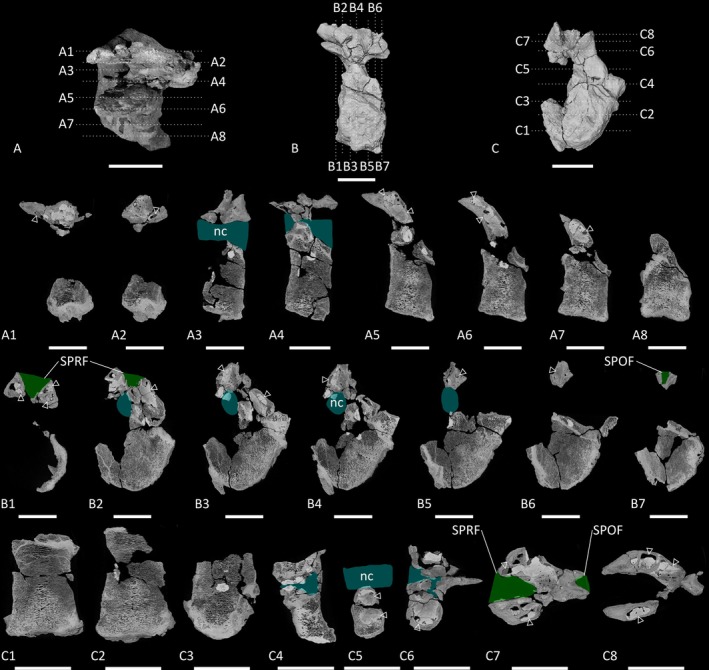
CT scan of *Wintonotitan wattsi* anterior caudal vertebra ‘D’ QMF7292. (A) photograph dorsal view, (B) 3D rendering left lateral view, (C) 3D rendering anterior view; (A1–A8) CT scan slices in parasagittal cross‐section, (B1–B7) CT scan slices in transverse cross‐section, (C1–C8) CT scan slices in horizontal cross‐section. Nc, neural canal; SPOF, spinopostzygapophyseal fossa; SPRF, spinoprezygapophyseal fossa. Key: Blue highlight, neural canal; green highlight, neural spine fossa; white arrow, pneumatic bone texture. Scale bars = 100 mm.
